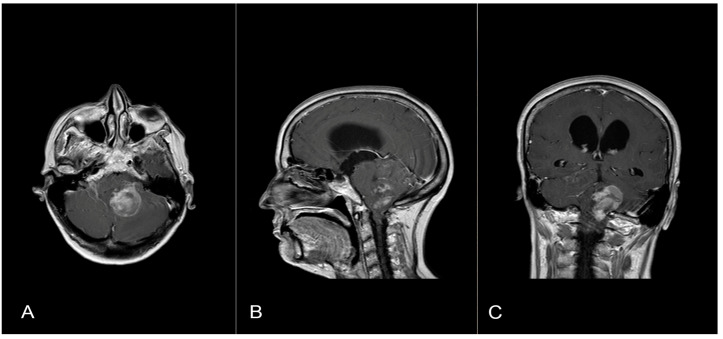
Axial (A), sagittal (B) and coronal (C) T1 contrast-enhanced weighted brain MRI shows a lesion in the left cerebellar hemisphere involving the middle-lower cerebellar pedicle surrounded by perilesional edema and tri-ventricular obstructive hydrocephalus for compression of the IV ventricle.
